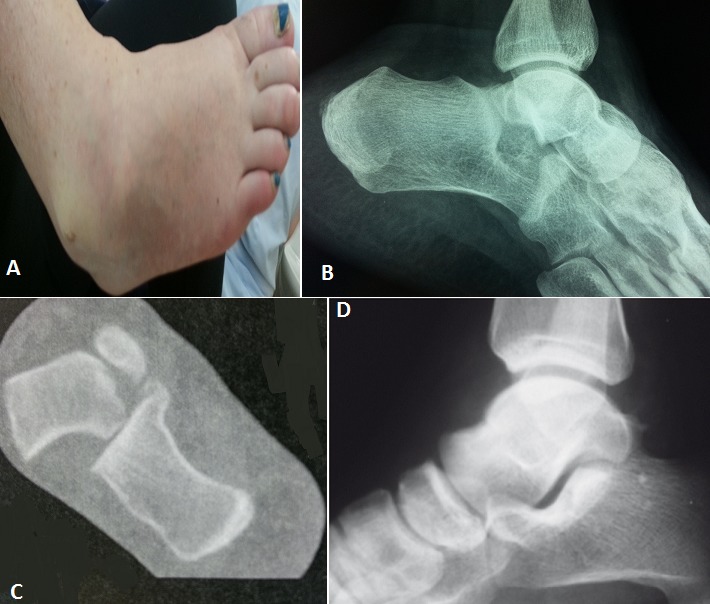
(A) image Clinique; (B) radiographie standard de profil de la cheville et du médio-pied; (C) scanner de la cheville et du pied confirmant la lésion; (D) contrôle radiographique de la réduction orthopédique avec une bonne congruence articulaire
